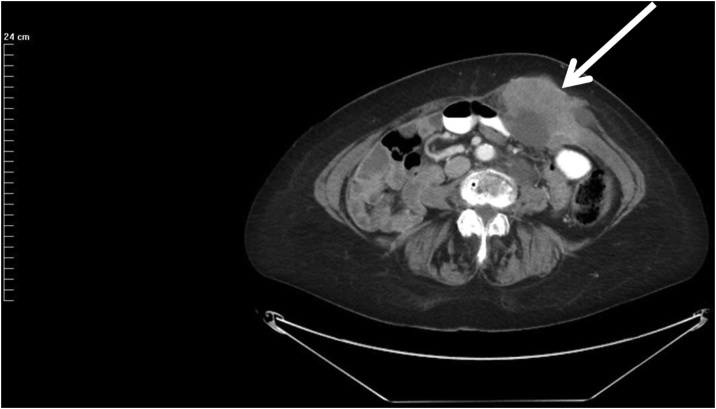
CT cut through the left mid-abdominal laparoscopy port site metastases. The entire thickness of the left rectus muscle appears to be infiltrated by serous ovarian cancer. The skin and subcutaneous tissue beneath the skin are not involved. The bowel directly beneath the mass does not appear to be invaded.
